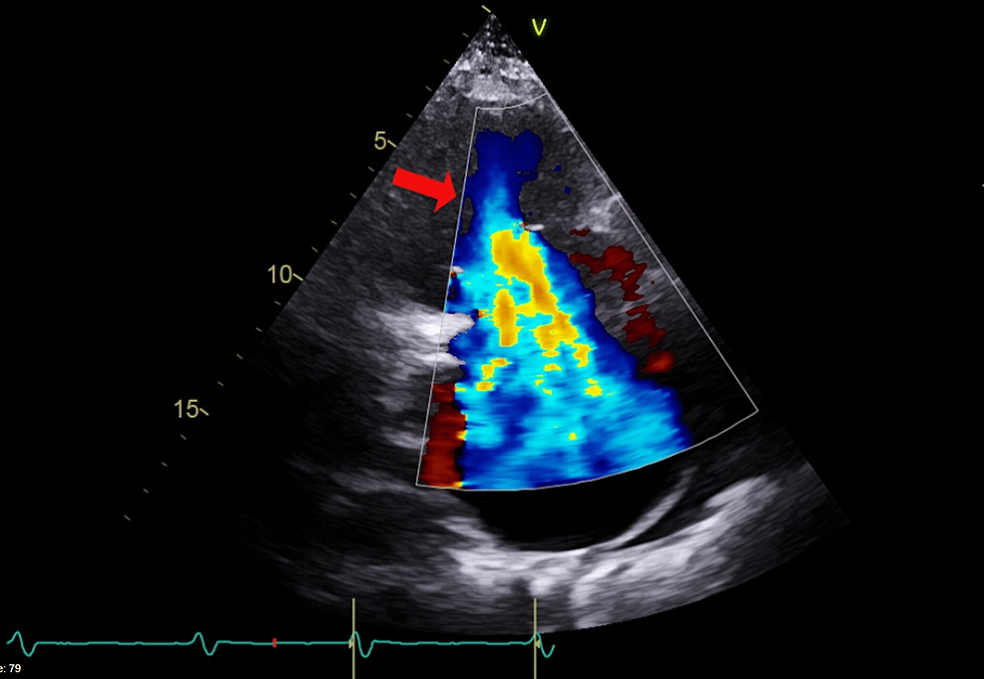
Echocardiogram (image 3) The image is showing tricuspid regurgitation (TR) (red arrow).
